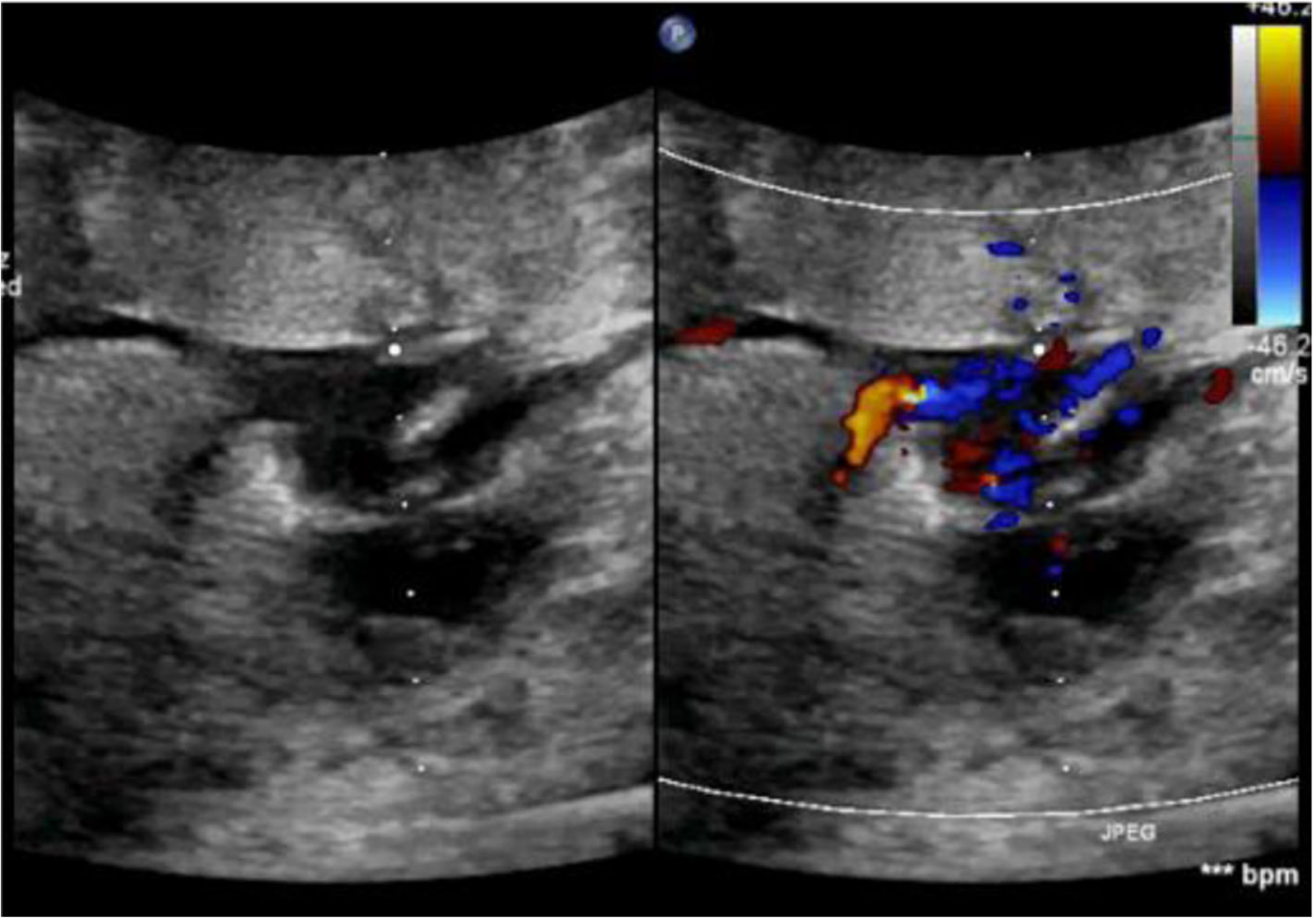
Normal systemic veno-atrial connection by both 2D and color Doppler imaging in a case 12-week gestation
